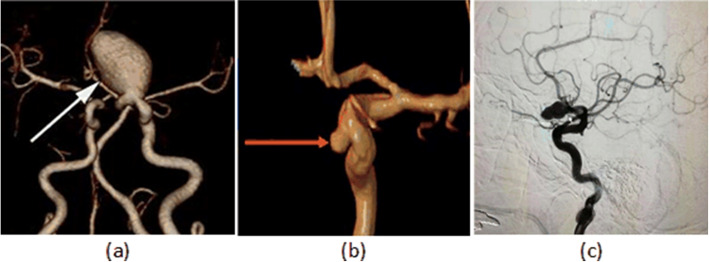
Clinical Imaging Examples of IAs. a CTA Example; b MRA Example; c DSA Example
